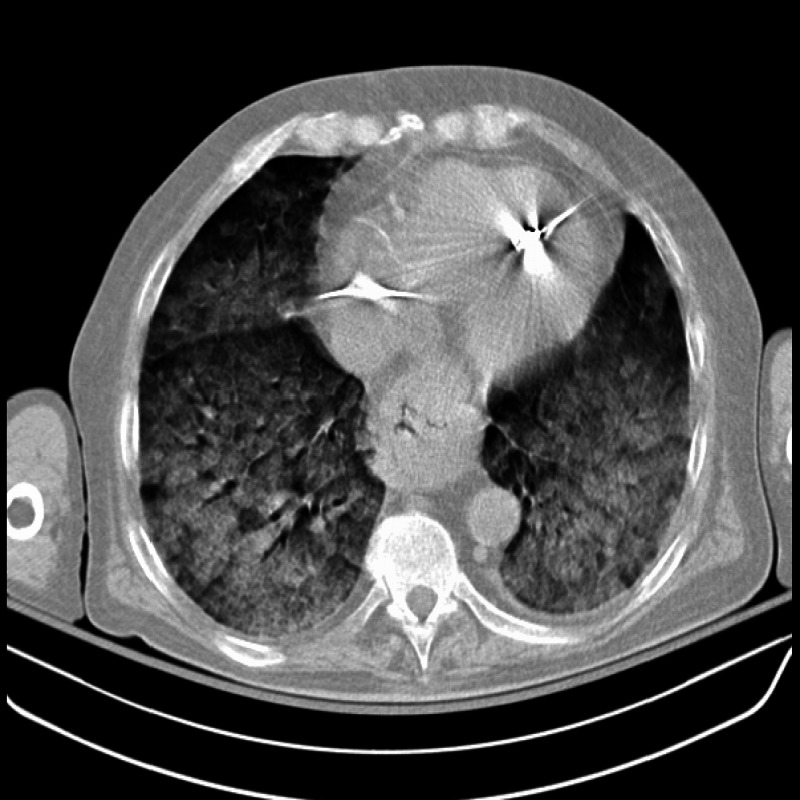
Chest CT scan indicative of severe ARDS. ARDS: Acute respiratory distress syndrome
